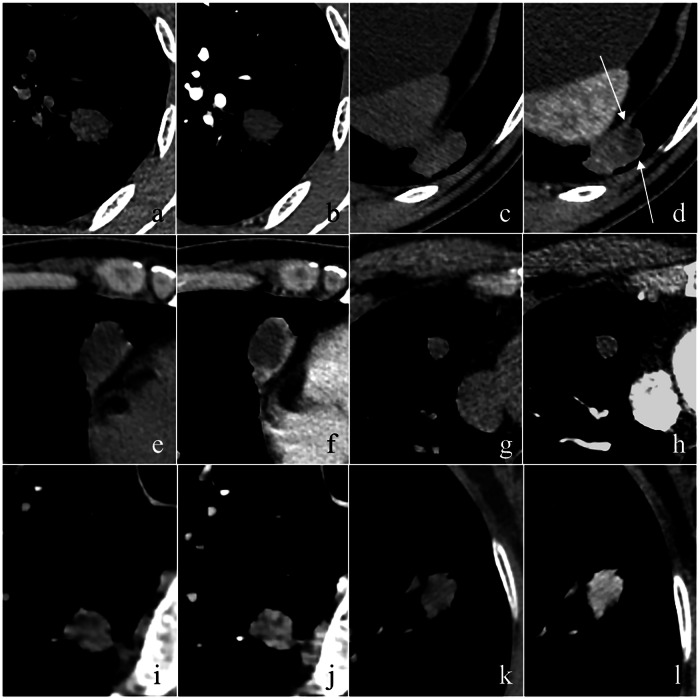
Enhancement patterns of nodules on CECT images with mediastinal window. A 52-year-old man with a tuberculous granuloma. A transverse non-enhanced CT image shows a round SN (12 HU) located in the left upper lobe (a), which has no significant enhancement (▵CT value = 0 HU) on the CECT image (b). A 40-year-old man with necrotizing granuloma. A transverse non-enhanced CT image shows an oval SN (38 HU) located in the left lower lobe (c), which has heterogeneous enhancement with well-defined necrosis (arrows) on the CECT image (d). A 50-year-old woman with a tuberculous granuloma. A transverse non-enhanced CT image shows a round SN (41 HU) located in the right lower lobe (e), which has rim enhancement on the CECT image (f). A 66-year-old woman with necrotizing granuloma. A transverse non-enhanced CT image shows a round SN (10 HU) located in the right upper lobe (g), which has mild and heterogeneous enhancement (▵CT value = 20 HU) on the CECT image (h). A 47-year-old woman with adenocarcinoma. A transverse non-enhanced CT image shows a round SN (26 HU) located in the right upper lobe (i), which has moderate and heterogeneous enhancement (▵CT value = 48 HU) on the CECT image (j). A 74-year-old woman with cryptococcal granuloma. A transverse non-enhanced CT image shows an oval SN (22 HU) located in the left upper lobe (k), which has severe and homogeneous enhancement (▵CT value = 86 HU) on the CECT image (l). CT, computed tomography; SN, solid nodule; CECT, contrast-enhanced computed tomography
